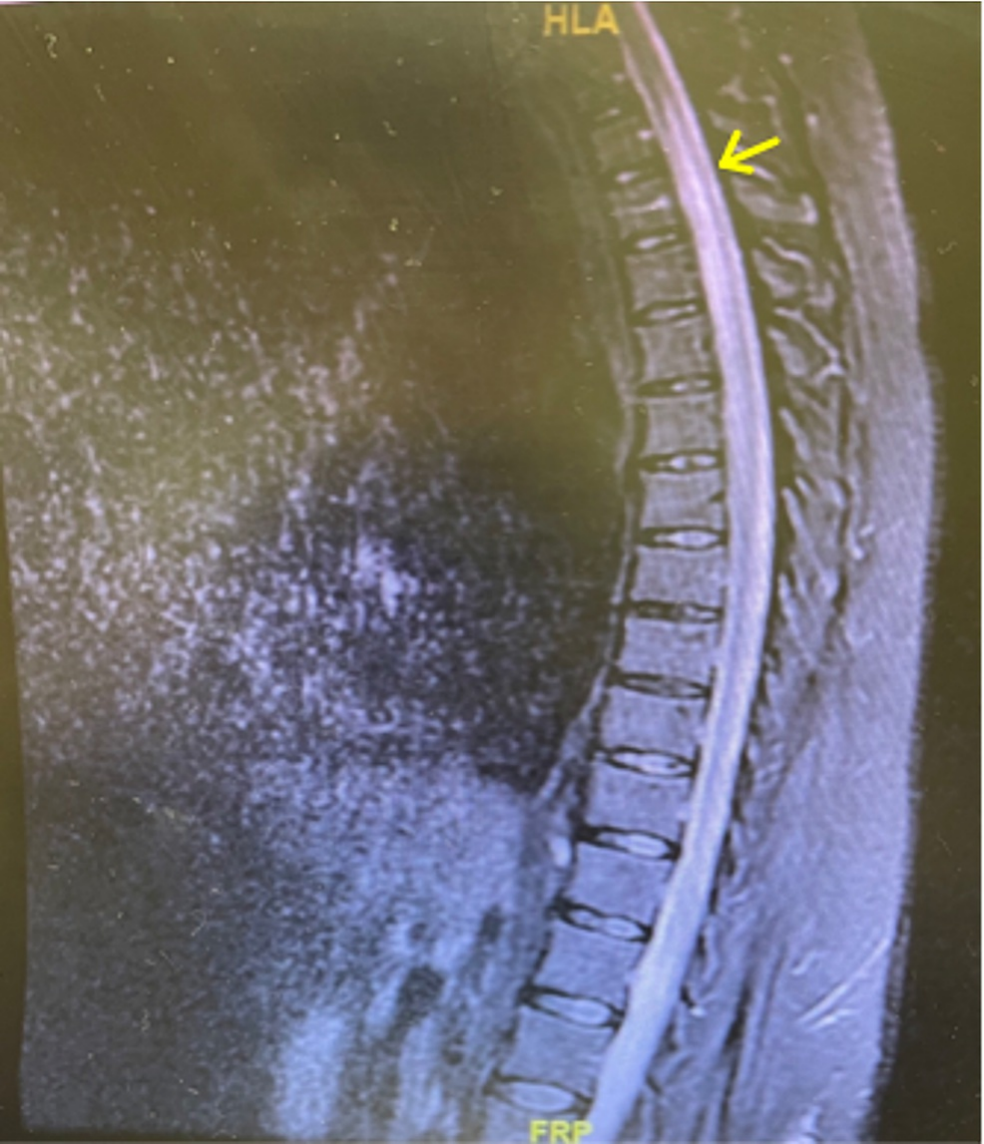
T2-weighted thoracic spine MRI showing hyper-intense intramedullary lesion from the level of C7 to T7 (yellow arrow).
